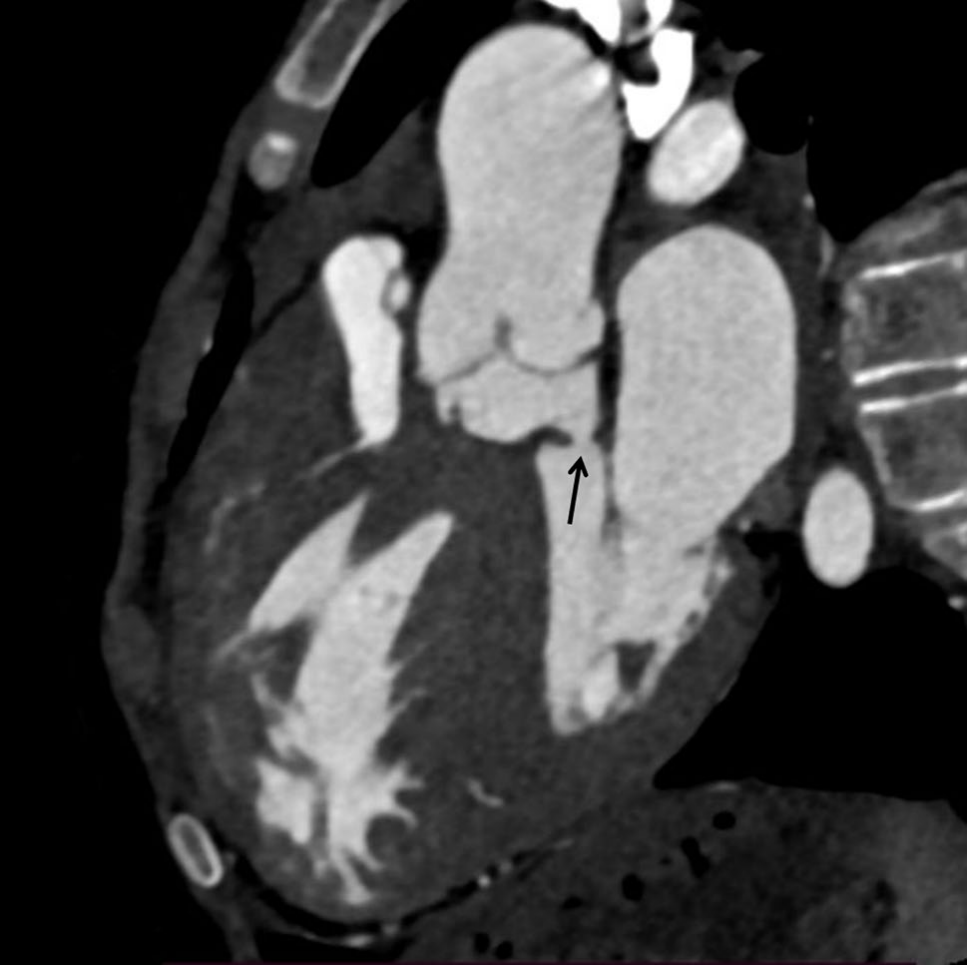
Cardiac CT oblique sagittal section showing subaortic membrane with a small fenestration (thin arrow)
